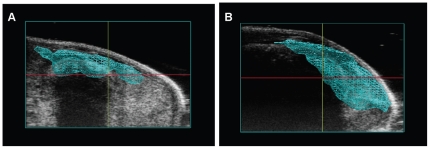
Representative ultrasound acquired images of orthotopic human pancreatic cancer xenografts 2 weeks after USGI of: A) MiaPaCa-2 cells B) SU86.86 cells. The blue shaded area represents the tumor generated by performing 3D tumor volume measurements.
